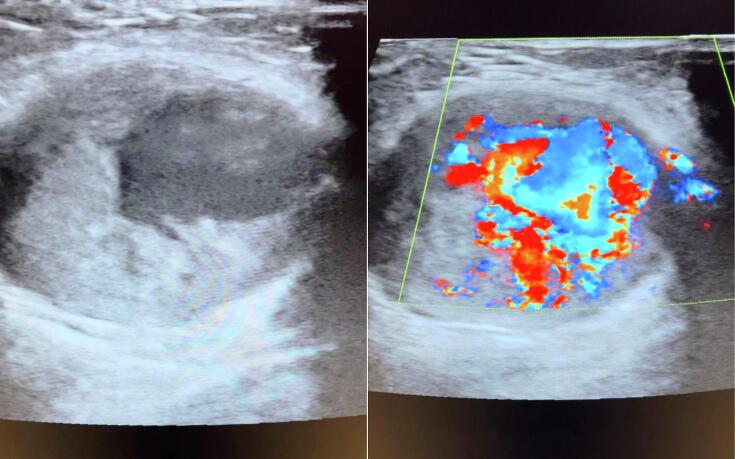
Gray scale and color Doppler sonography indicated a pulsating aneurysm with central regions of turbulent flow and peripheral solid thrombosis.
